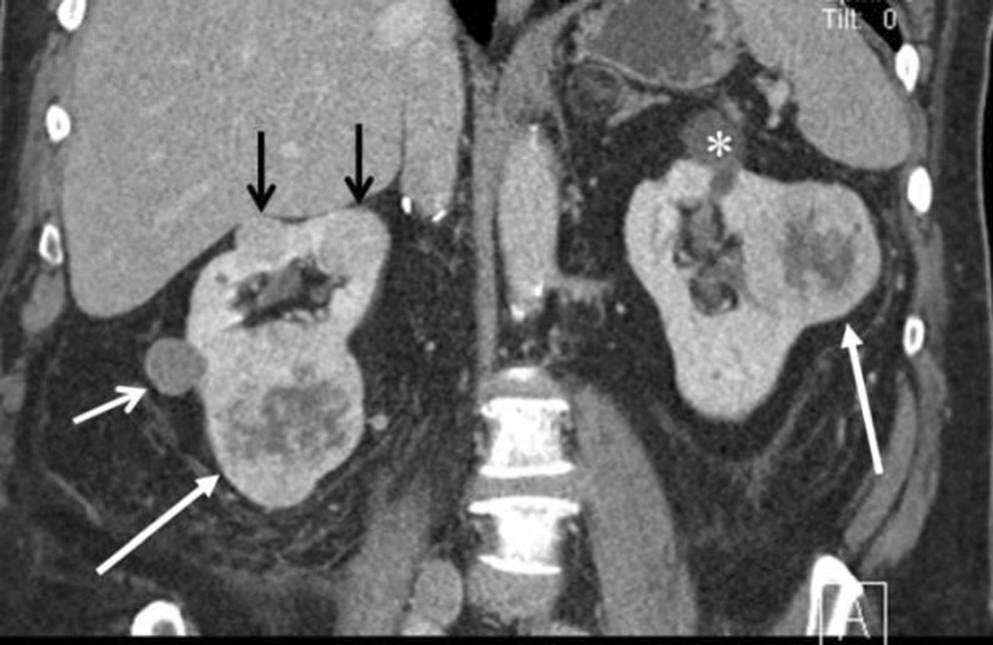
A 64-year-old man with bilateral multifocal oncocytomas coexisting with papillary RCC of the right kidney. The coronal reformatted image in the nephrographic phase of the CE-CT demonstrates five solid masses (*arrows*) and one simple cyst (*asterisk*). An exophytic right renal mass with relatively decreased contrast enhancement (*smaller white arrow*) was found to be papillary RCC. The four remaining enhancing masses were oncocytomas. Note that the small tumours are homogeneous (*smaller black arrows*), while the larger tumours are relatively heterogeneous (*larger white arrows*)
